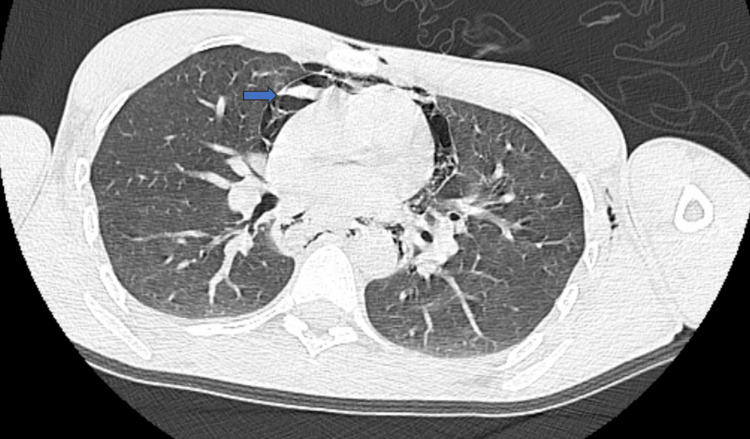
Axial chest CT image showing pneumomediastinum. The arrow highlights free mediastinal air.
